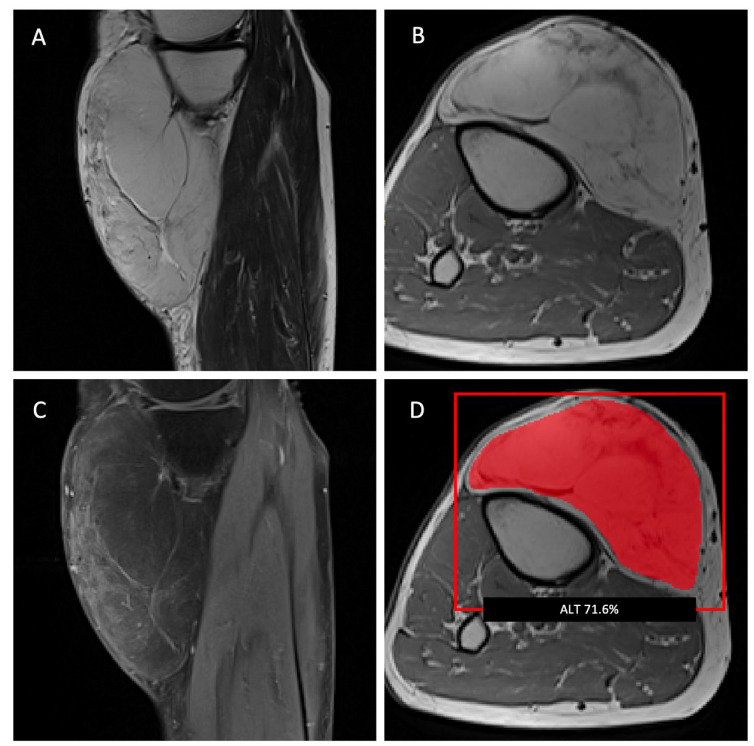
Sagittal T2-weighted (A) and axial T1-weighted (B) MR images of a lipomatous tumor located subcutaneously, anteromedial to the right proximal tibia. (C) A sagittal T1-weighted image with fat saturation shows a moderate septal contrast enhancement. All radiology residents classified this tumor as a lipoma, while the attending radiologist classified this tumor as an ALT. (D) The machine-learning algorithm also classified this tumor as an ALT with a probability of 71.6%. The diagnosis of an ALT was confirmed by pathology after surgical resection.
